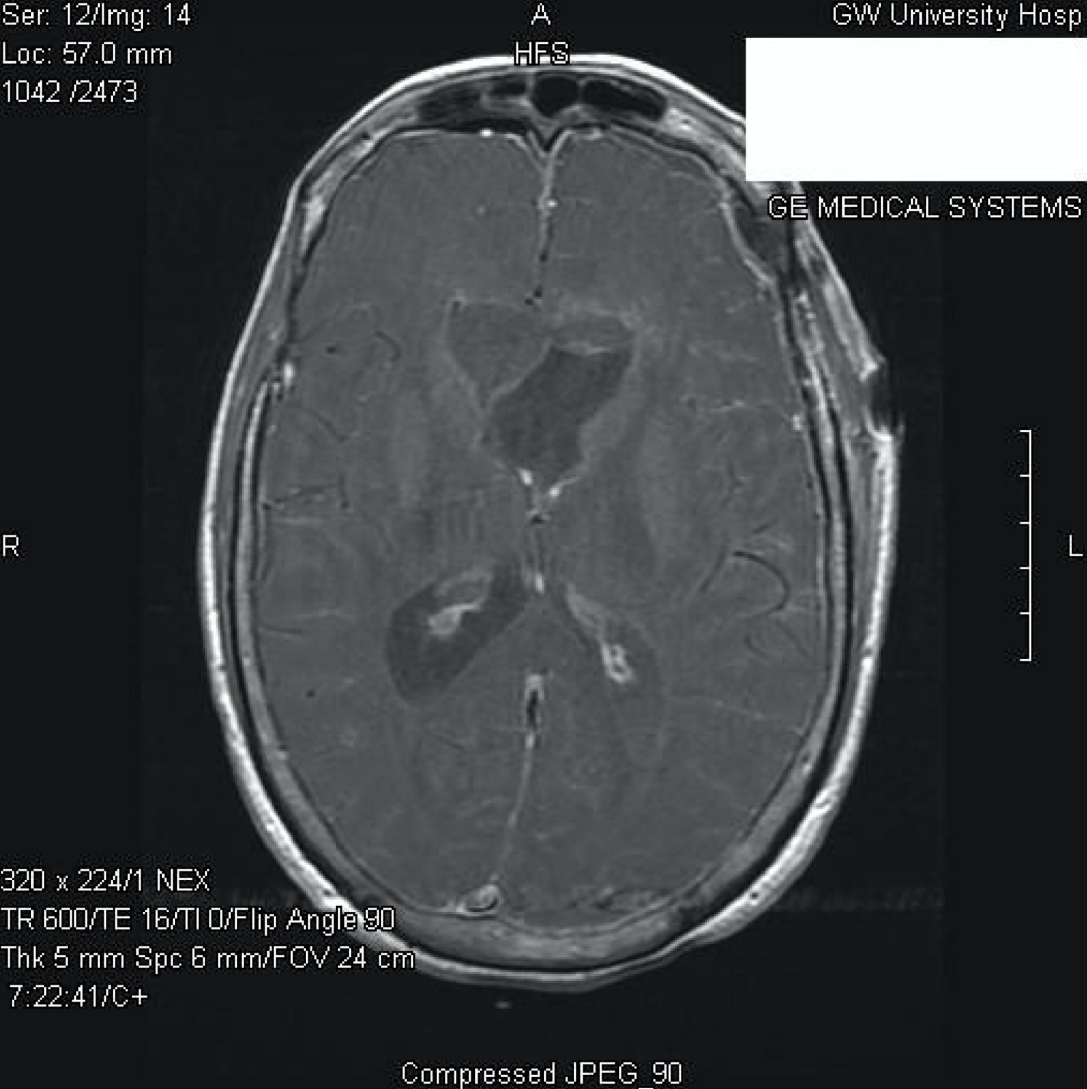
Postoperative axial post-contrast T1-weighted MR image MR: magnetic resonance
